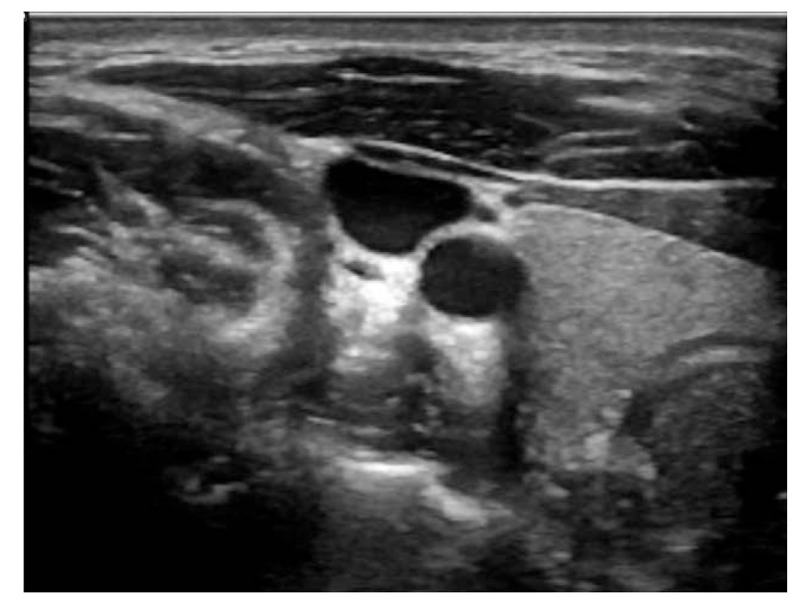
Intraoperative ultrasound of left arm showing no Nexplanon.
